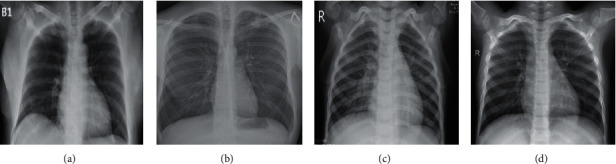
X-ray image samples of the 4 classes: (a) COVID-19 sample; (b) tuberculosis sample; (c) viral pneumonia sample; (d) normal sample.
